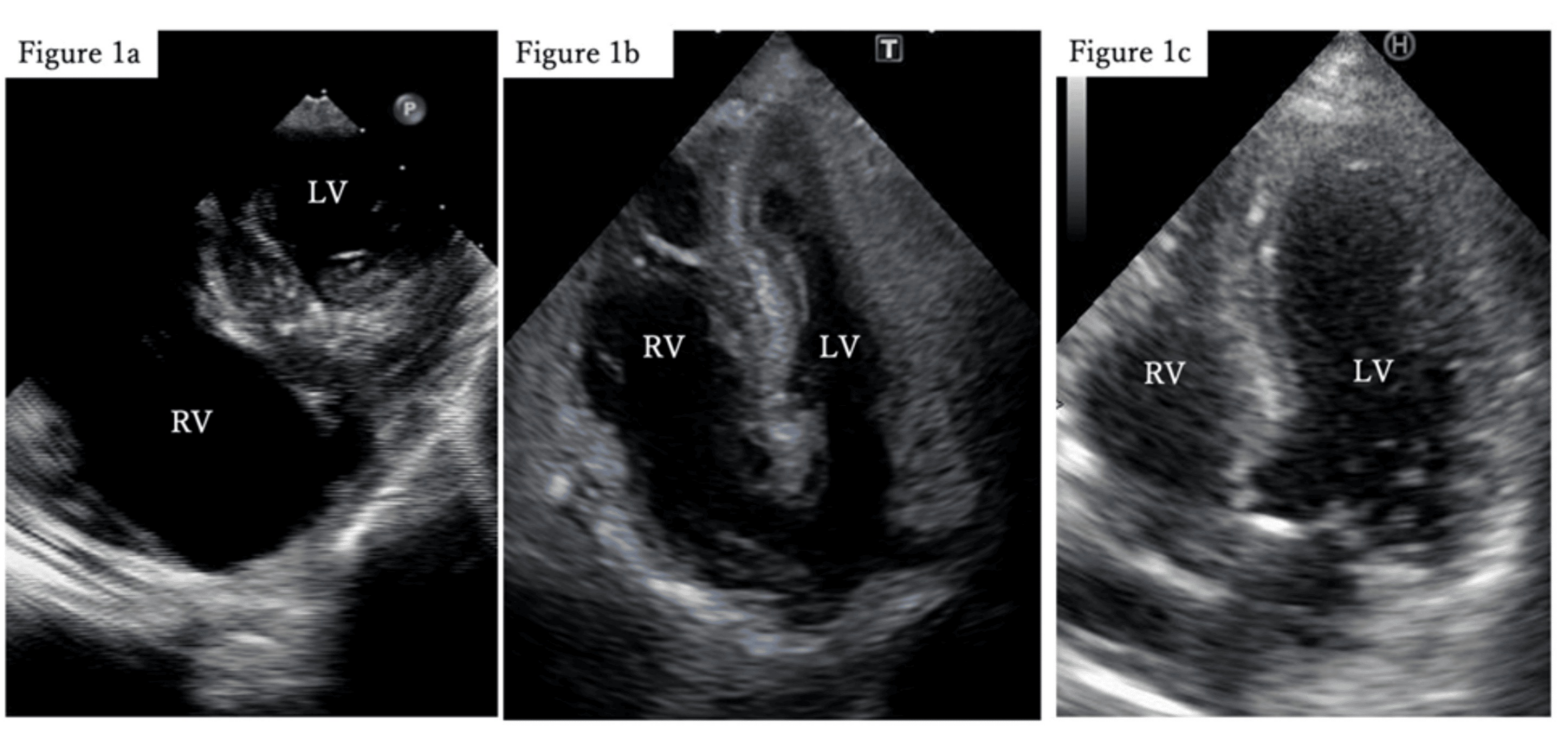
Transesophageal and transthoracic echocardiography RV=right ventricle, LV=left ventricle, VA-ECMO=veno-arterial extracorporeal membrane oxygenation, iNO=inhaled nitric oxide 1a: Transesophageal echocardiography (transgastric mid-short-axis view) during acute shock; 1b: Transthoracic echocardiography (apical four-chamber view) after initiation of VA-ECMO; Figure 1c: Transthoracic echocardiography (apical four-chamber view) after discontinuation of iNO.
